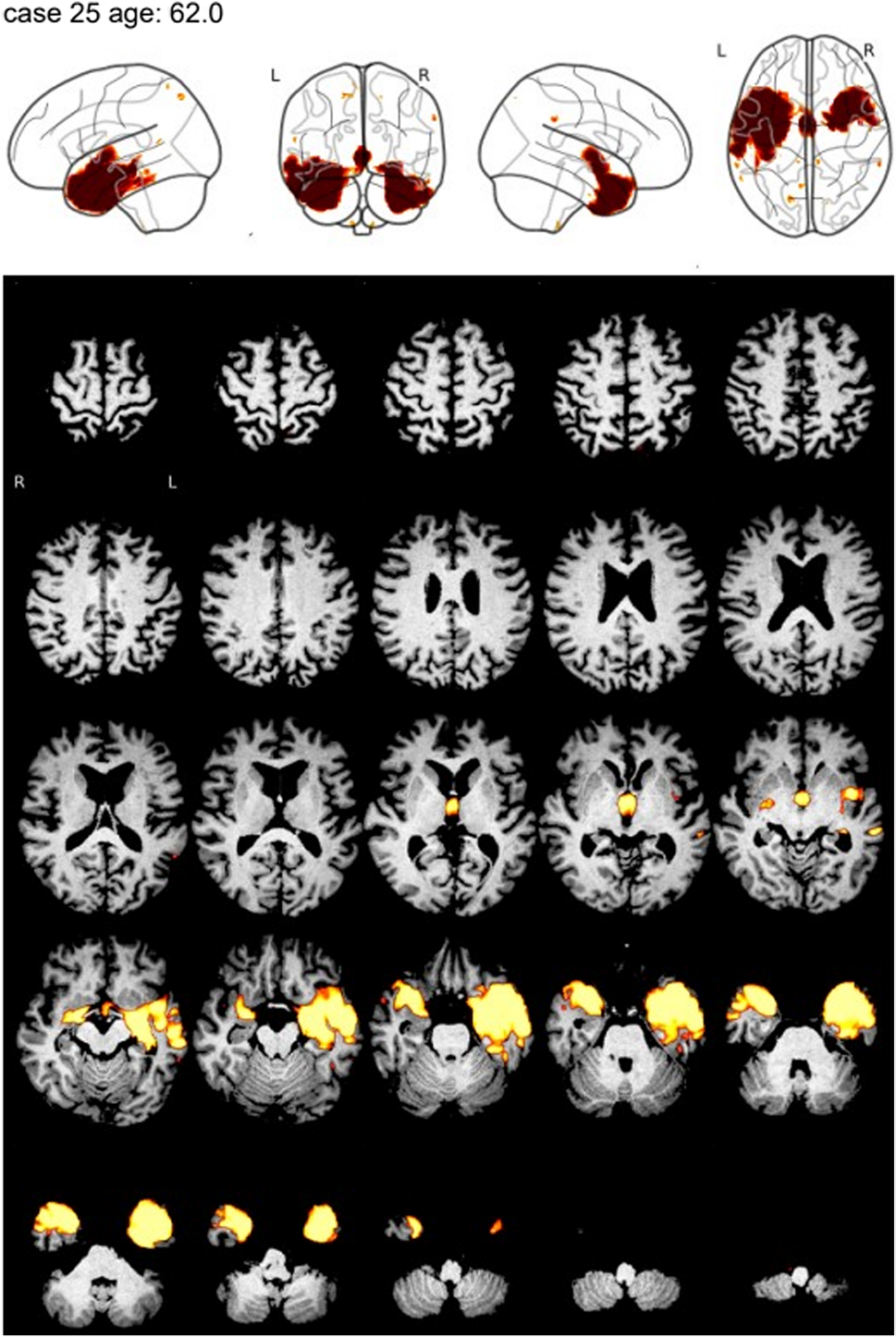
Standardized display for visual interpretation of the thresholded VBM map. The display combines transversal slices of the thresholded VBM map overlaid to the individual T1w-MRI scan and a glass brain view of the thresholded VBM map in a single-page pdf document. The example shows the CNN-VBM map of a patient with semantic variant primary progressive aphasia
